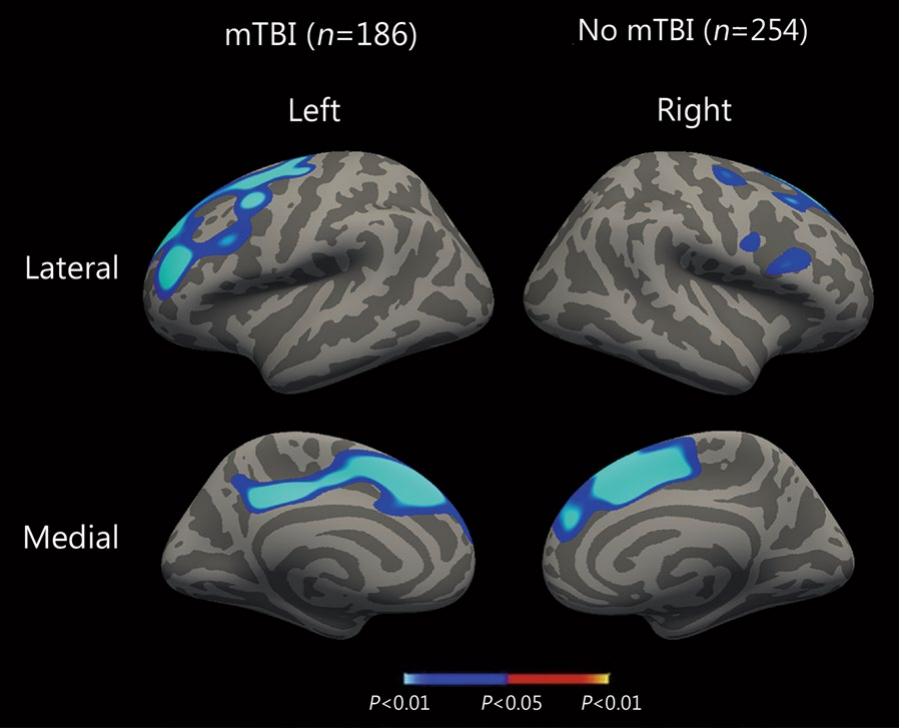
Magnetic resonance imaging (MRI) maps showing interactive effect of mild traumatic brain injury (mTBI) and Pittsburgh Sleep Quality Index (PSQI) on cortical thickness. Blue areas indicate significantly decreased cortical thickness with increased PSQI in individuals with mTBI (*n* = 186) compared to individuals without mTBI (*n* = 254), corrected for age, sex, Clinician-Administered post-traumatic stress disorder (PTSD) Scale and Depression Anxiety Stress Scale—Depression Subscale, and comorbid PTSD
